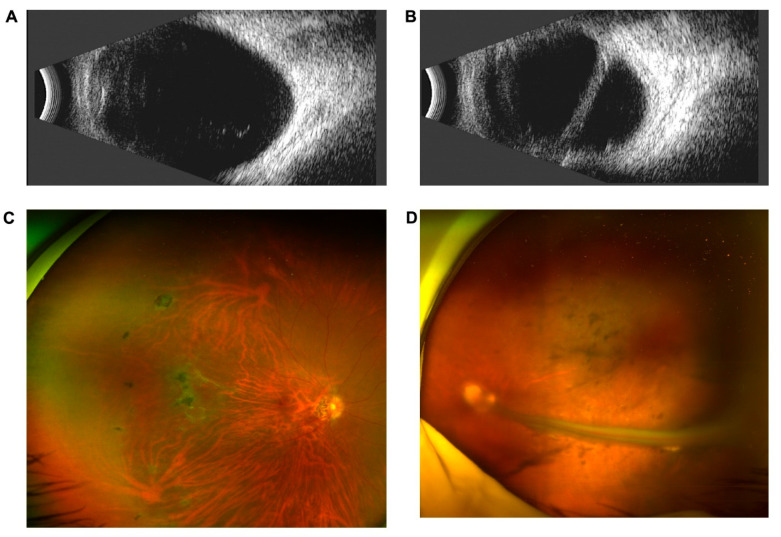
Clinical features of DX1344. B-scan ultrasonography showed vitreous opacity in the right eye (A) and retinal folds in the left eye (B). Scanning laser ophthalmoscopy showed pigmentation on the nasal retina of the right eye (C) and retinal folds in the left eye (D).
